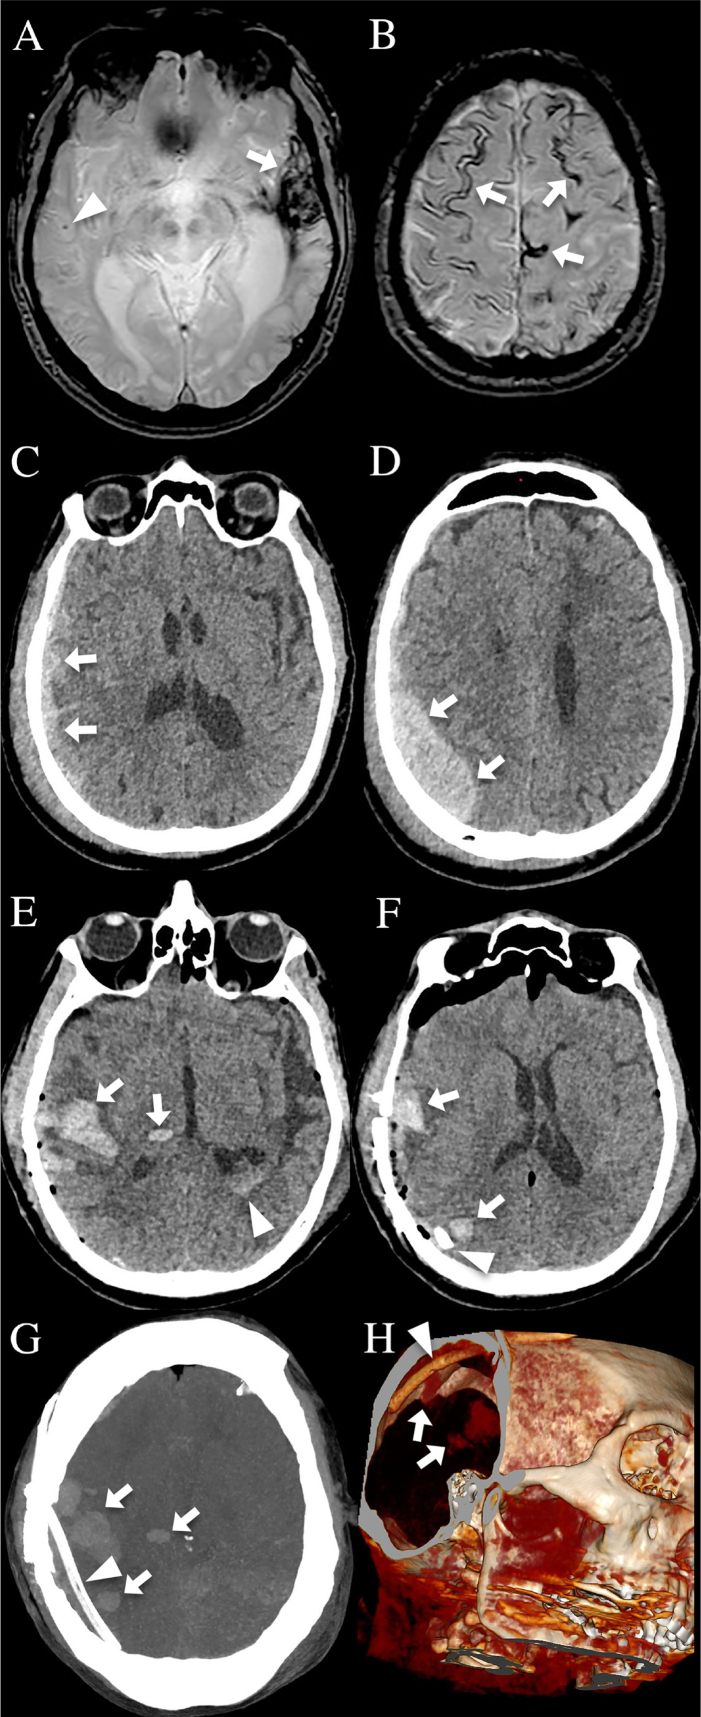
CAA patient 1 showing chronic ICH (A, arrow), cerebral microbleed (A, arrowhead), and cortical superficial siderosis (B, arrows) on MRI performed six months before head trauma. After major blunt head trauma, sub- (C) and extradural (D) hemorrhage can be observed on CT. After hemorrhage evacuation, a subdural drain (F, G, and H, arrowhead) was inserted complicated by multiple lobar and deep ICH intracerebral (E-H, arrows) in brain regions earlier compressed by the initial extra/subdural hemorrhage and in front of the subdural drain (E and F are standard CT images, G 2D and H 3D multiplanar reconstruction CT images) together with intraventricular hemorrhage (E, arrowhead) on postoperative CT.
